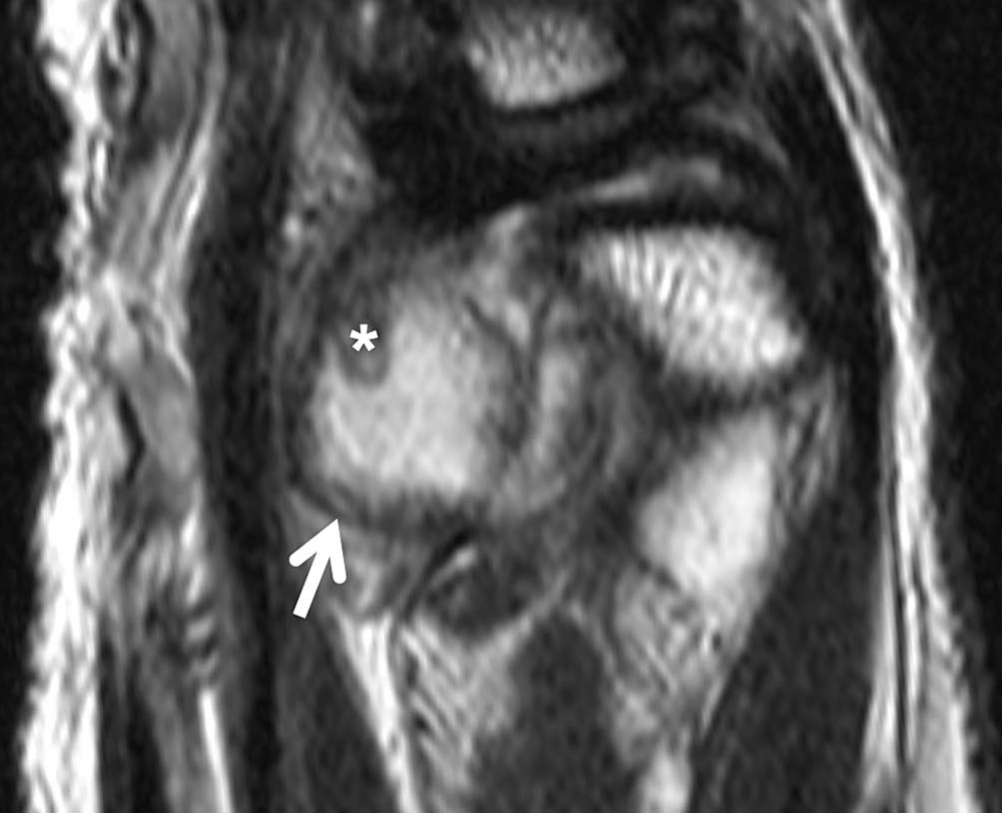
Sagittal slice of a T2-weighted fast-spin echo sequence of the DRUJ—well-defined hyperintense lesion on the volar aspect of the DRUJ; capsular blooming artifact (short arrow); hypointense, internal structure within the distended DRUJ (asterix), chondromatosis foci accordingly.
